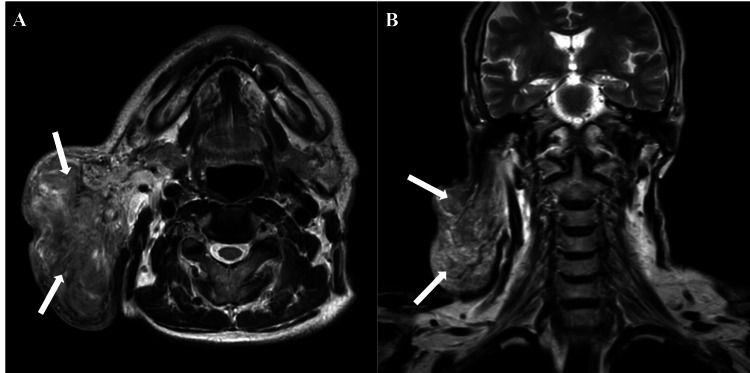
MRI findings Axial (A) and coronal (B) T2-weighted images showing a homogeneous, well-defined mass in the right parotid gland extending posteriorly but without deep tissue invasion or neurovascular involvement. MRI: magnetic resonance imaging
